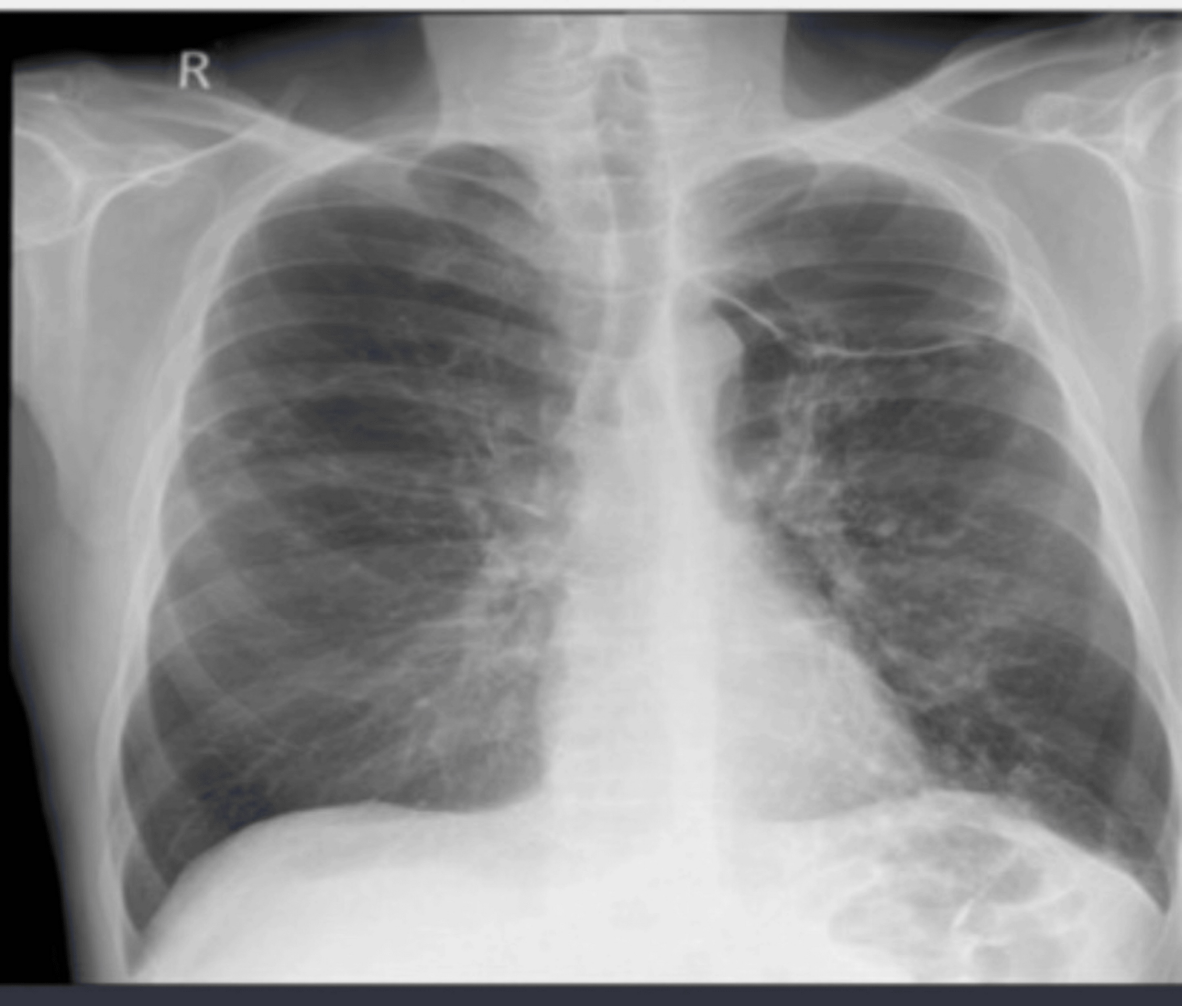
Chest AP erect plain film The image shows significant improvement in the appearance of the lung cavity.
